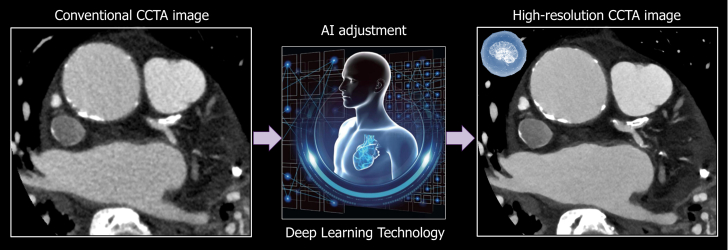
AI-Enhanced High-Resolution CCTA Comparison of conventional CCTA and AI-enhanced CCTA images. AI adjustment using the Precise IQ Engine (PIQE) algorithm resulted in superior spatial resolution. AI = artificial intelligence; CCTA = coronary computed tomography angiography.
